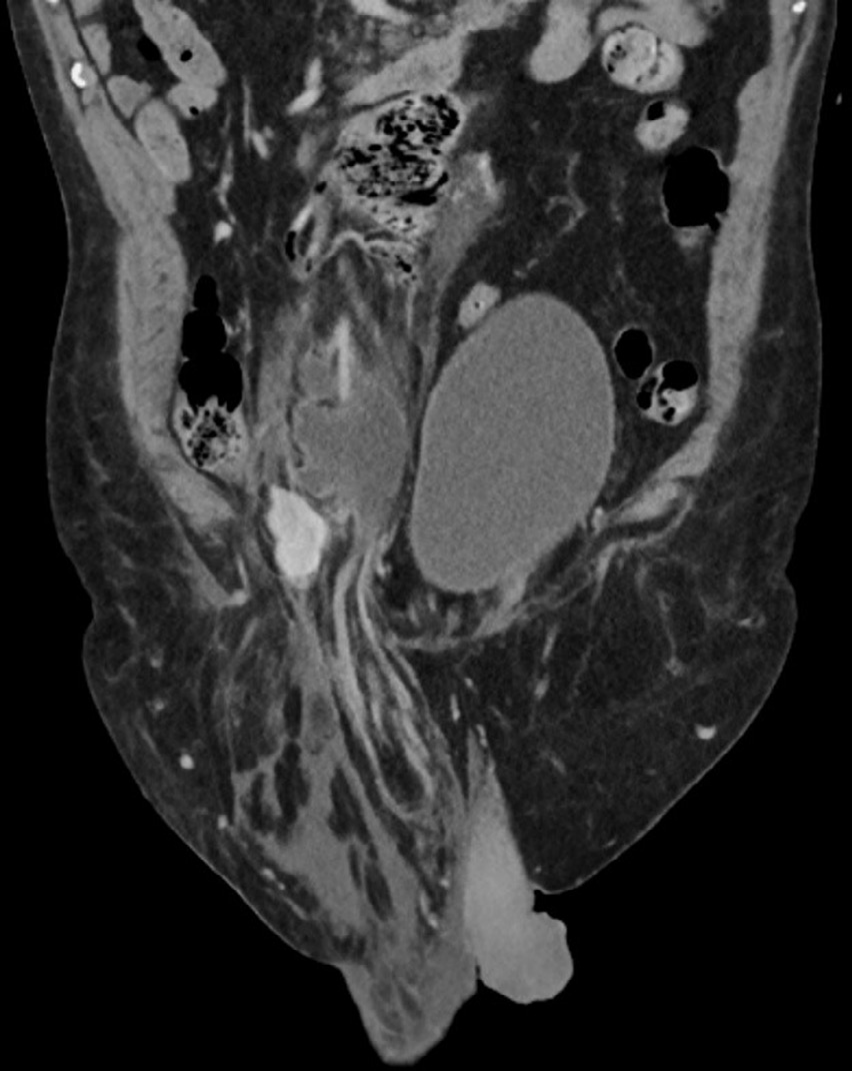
CT aortogram from 2019 showing the right ureter coiled within the hernial sac.
